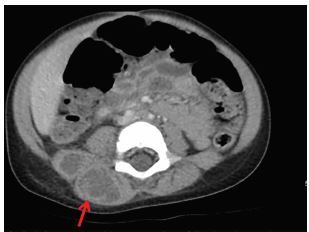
Axial computed tomography of the lumbar spine revealing areas of low density with some rim enhancement in the right posterior paraspinal muscles (arrow) consistent with abscess.
